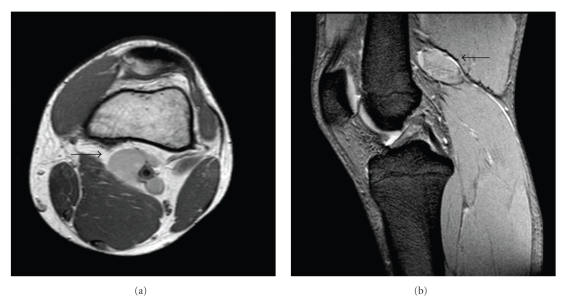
Magnetic Resonance* (MRI), SE T1 Dual axial (a), SE T2 FFE sagittal (b) showing a 4 cm oval-shaped formation with dishomogeneous hyperintensity in the left popliteal fossa adjacent to the vessels.
